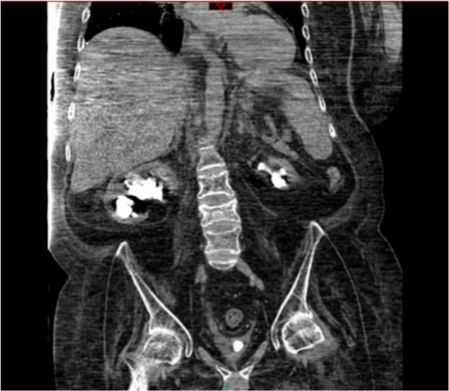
Coronal section of CT performed prior to lithotripsy: Both kidneys normal in size; bilateral staghorn calculi larger on the right side; no hydronephrosis; empty urinary bladder with bladder stone measuring 1.5 cm in diameter.
